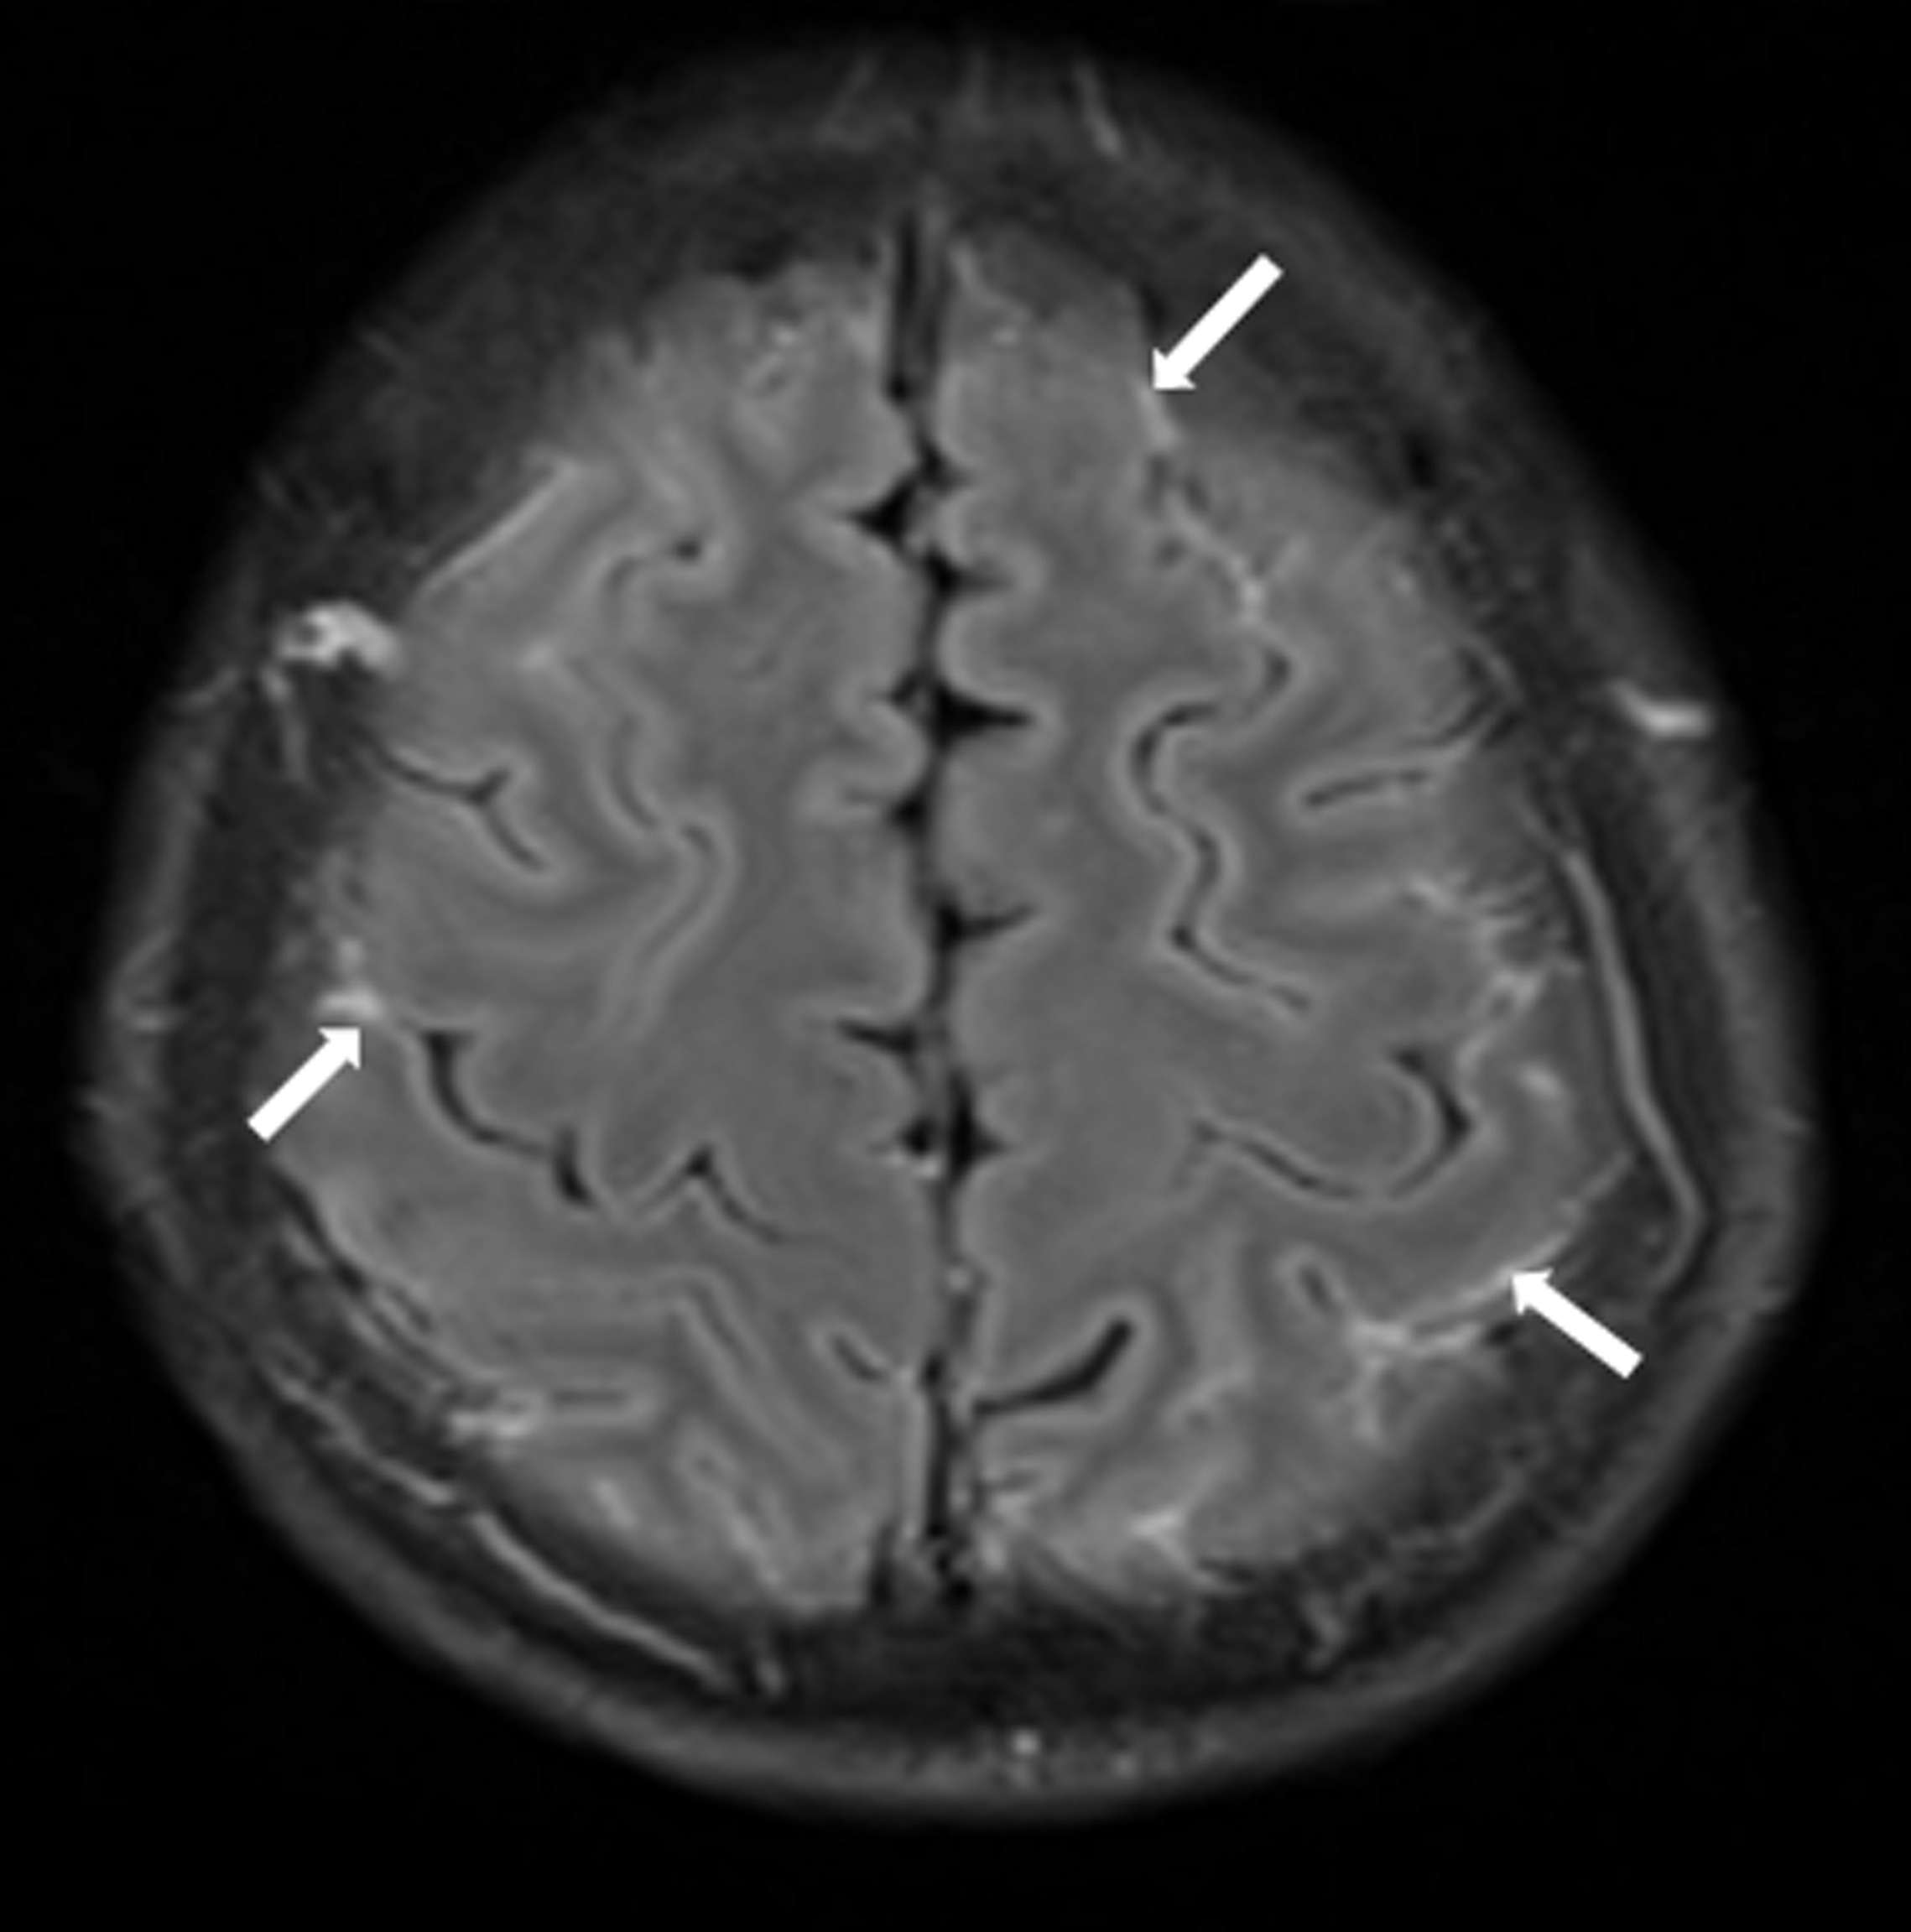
Magnetic resonance image of the brain. Abnormal hyperintensity in the cerebral sulci of the bilateral frontal-parietal lobes can be observed on fluid-attenuated inversion recovery sequences with gadolinium enhancement
